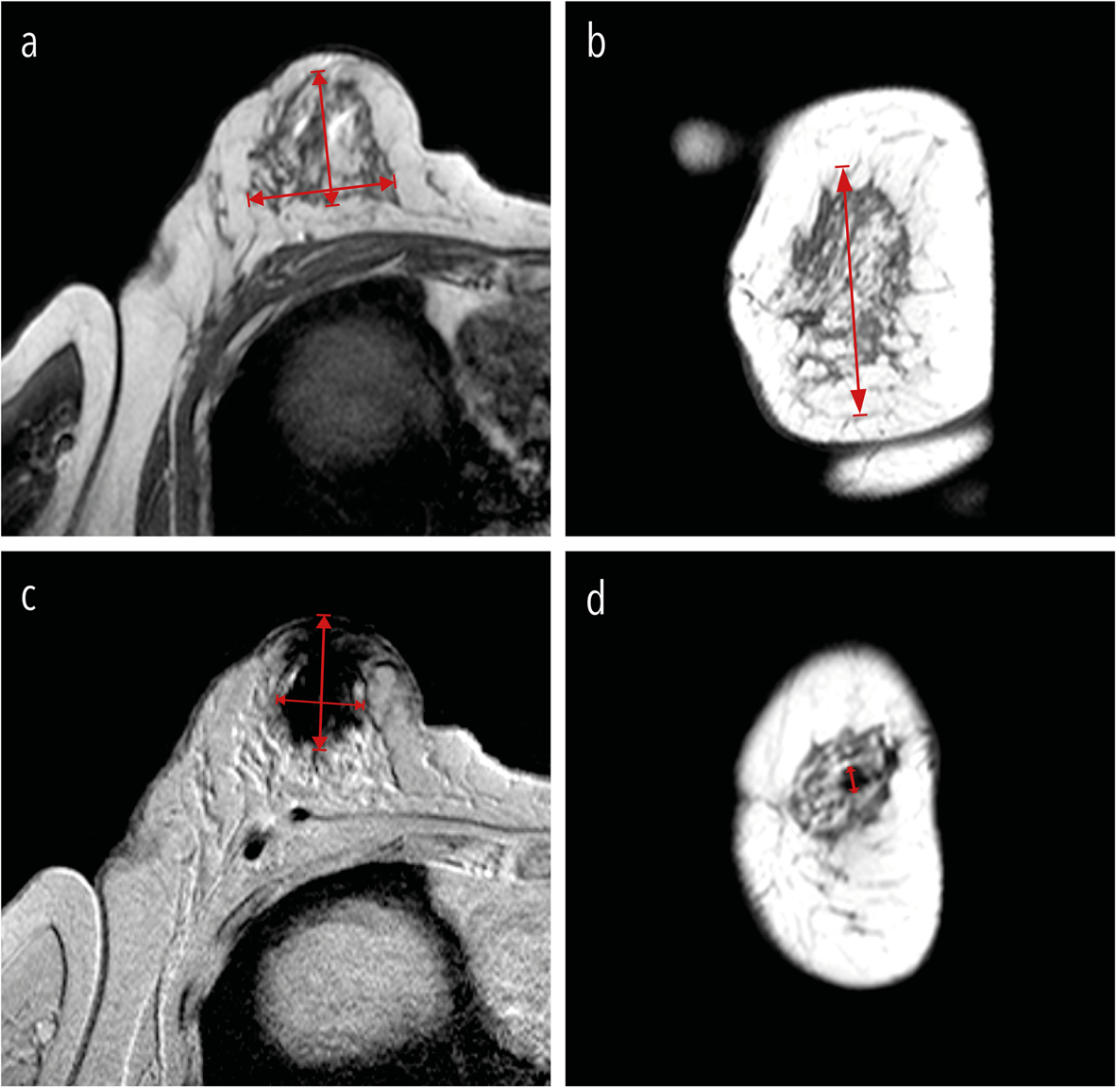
FGT and artefact measurements. The overall volume of FGT was manually assessed by both radiologists independently on T1-weighted images in three orthogonal planes. Dg_1_ and Dg_2_ were the maximal extension measured in the axial plane (a). Dg_3_ was the maximal extension measured in the coronal plane (b). The artefact diameter assessed on both T1- and T2*-weighted images in three orthogonal planes are shown in c (Da_1_, Da_2_) and d (Da_3_)
